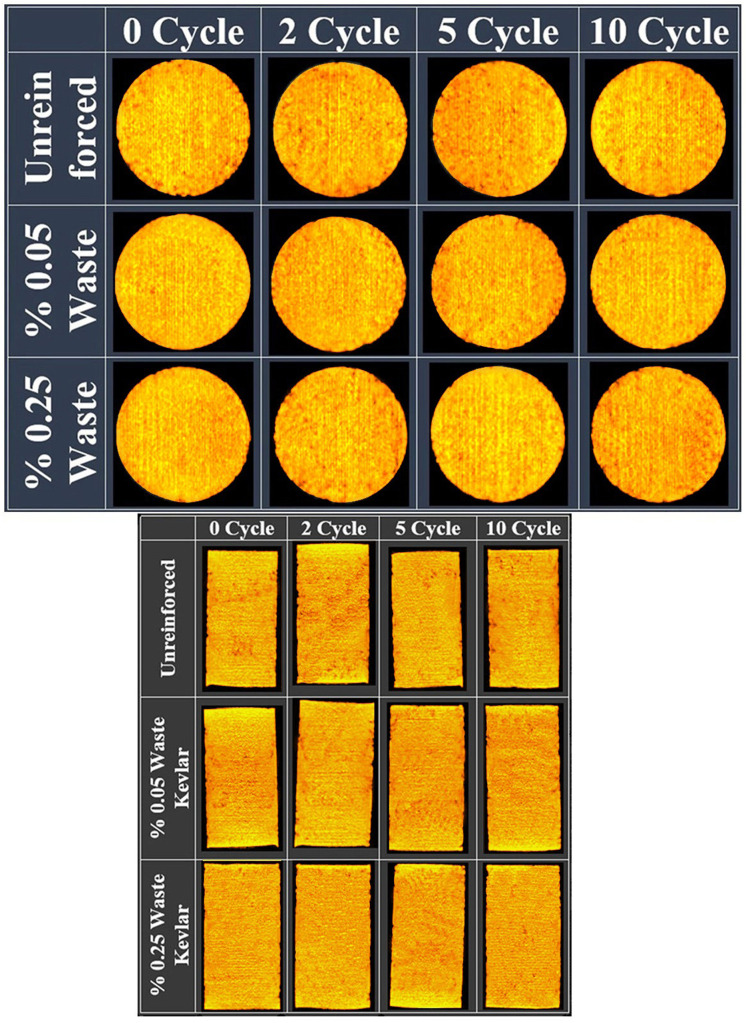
CT scan of CH clay (a- The top of sample’ 3 mm cross section image b- The middle of sample’ longitudinal section image).
